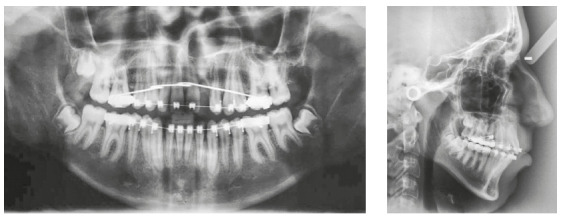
Radiographies during the progress of treatment.
